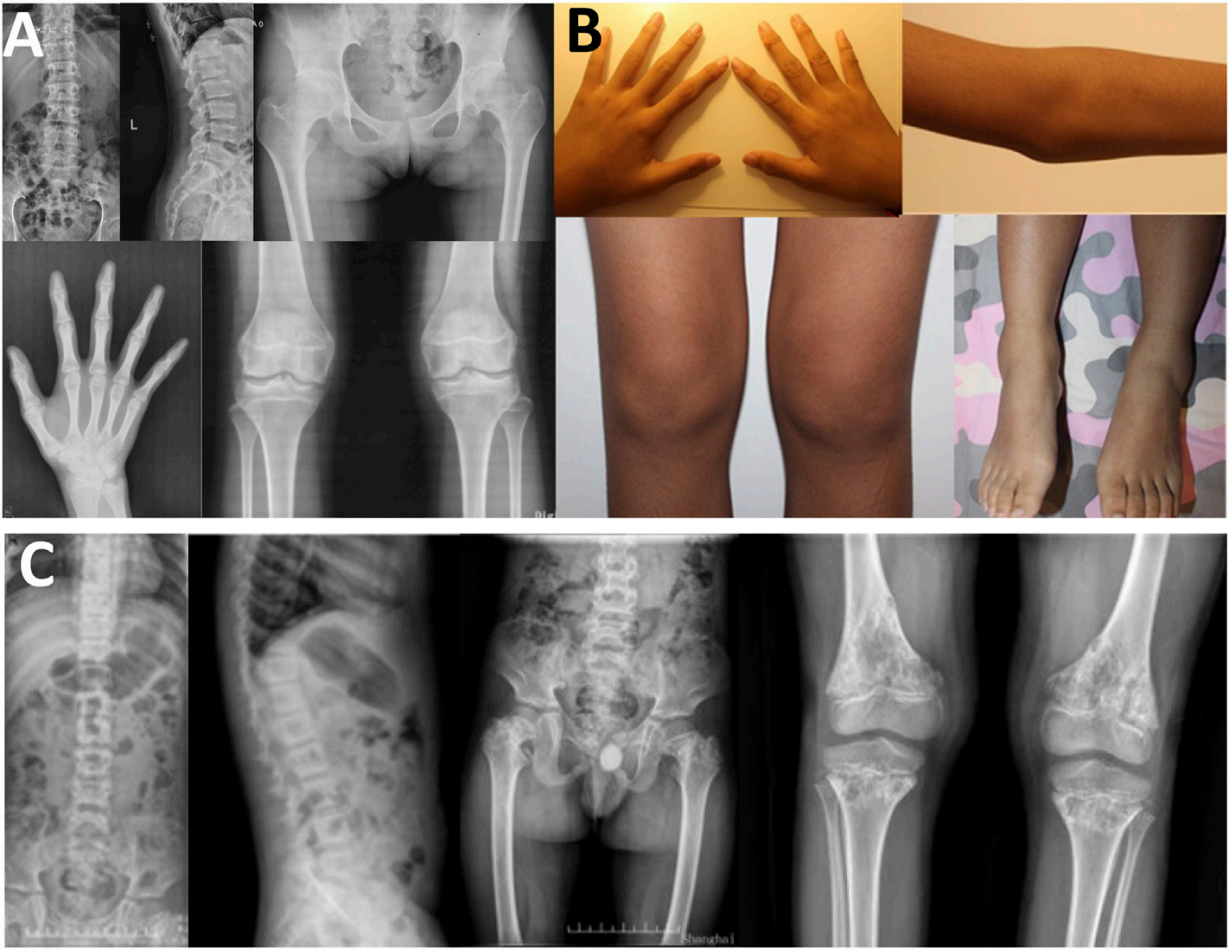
Photographs and radiographs of the families 5 and 6. (A,B) Radiographs of the spine, pelvis, hands, knees (A) and photographs (B) of proband 5 (Ⅱ-1) in family 5. (A) Mild platyspondyly was revealed in the spine. The pelvis was normal. Expansion of metaphysis and stenosis of joint spaces were visible in hands. Widened epiphysis and joint cavity stenosis were displayed in the knees. (B) Bilateral elbow, knee, ankle, and multiple metacarpophalangeal and interphalangeal joints were enlarged. (C) Radiographs of the spine, pelvis, and knees of proband (Ⅱ-1) in family 6. Irregular shape of the vertebral bodies was significant in the spine. Flattening of the acetabular roof, shortening of the femoral neck, mildly compressed femoral head, and thickening of metaphyseal texture were displayed in the pelvis. Widened epiphysis and metaphysis, irregular shape of the epiphysis, and disorganization of the metaphysis were obvious in the knees.
